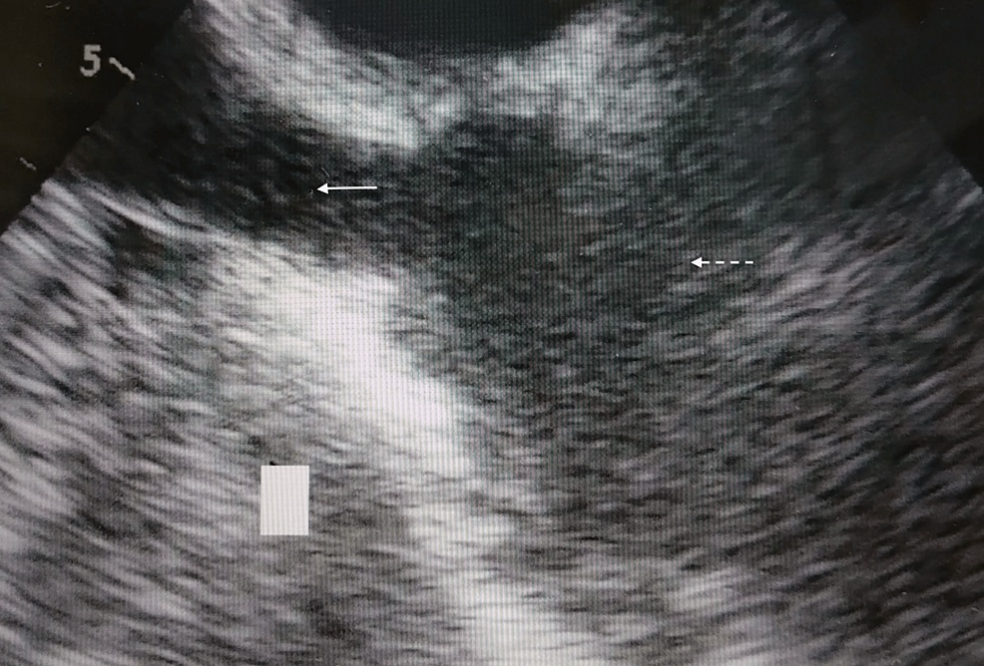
Bicaval transesophageal echocardiography views after insertion of the Power PICC in Case 4. The white arrow shows the superior vena cava, and the white dotted line shows the right arial.
